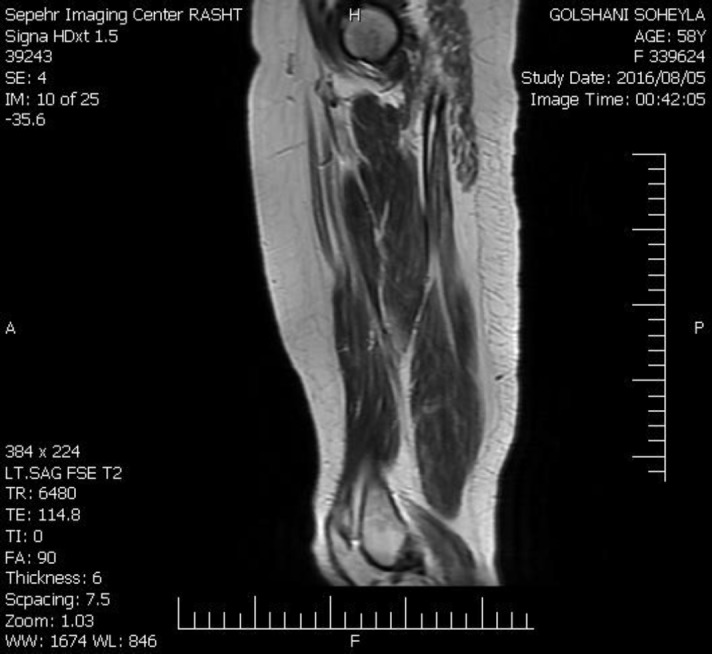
MRI of thigh. Signal alteration (low on T1) with feathery appearance of muscle fibers.
